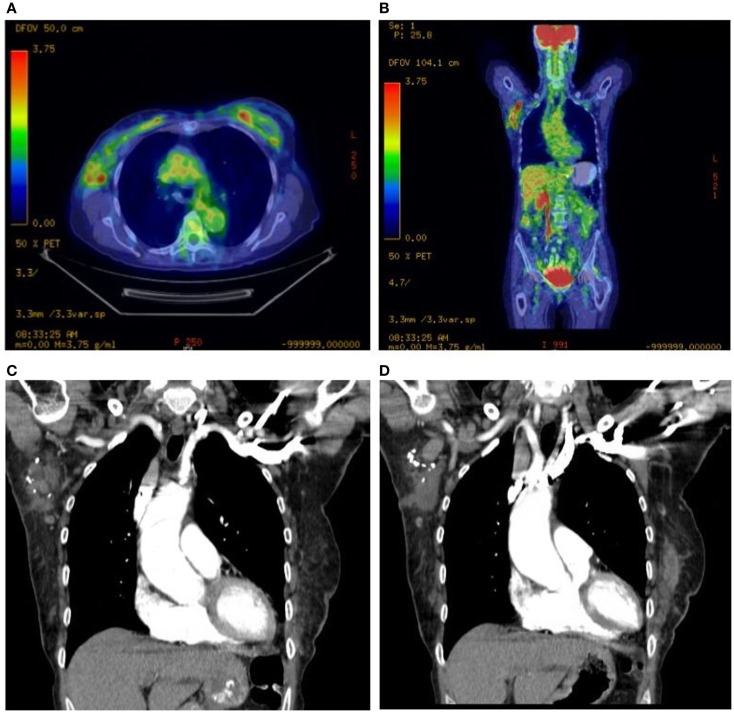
Right axillary lymphadenopathy demonstrated on axial and coronal PET scan images with abnormal FDG avidity (A,B) and coronal CT images with contrast (C,D).
